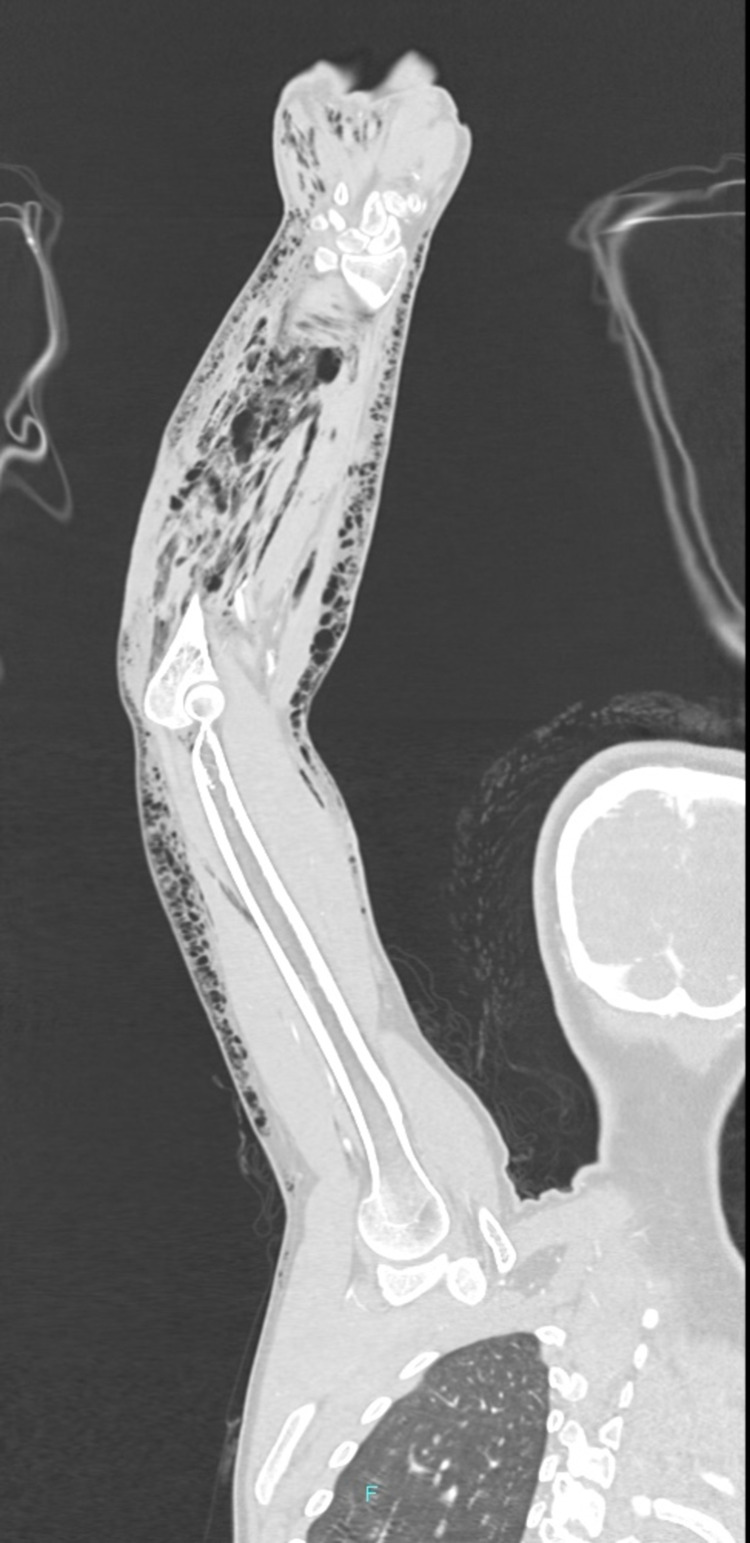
CTA of the right upper limb CTA of the right upper limb shows extensive emphysema and edema in the entire right upper limb in subcutaneous and deep soft tissues. Right brachiocephalic trunk, subclavian, axillary, brachial, radial, and ulnar arteries are grossly patent with no stenosis or malformation. CTA - computed tomography angiography
